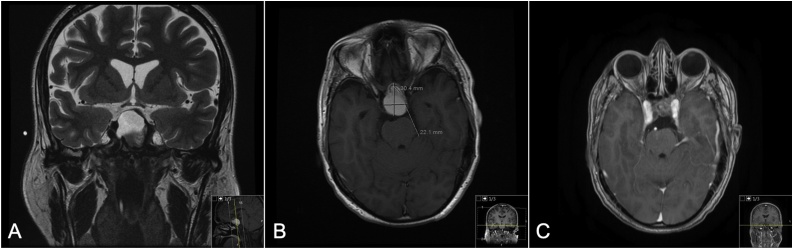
A: Pre-operative MRI T2 coronal section showing sellar/suprasellar lesion. B: Pre-operative MRI T1 with contrast axial section showing sellar/Suprasellar lesion. C: Nine months post-operative MRI T1 with contrast axial section with residual calcification.
